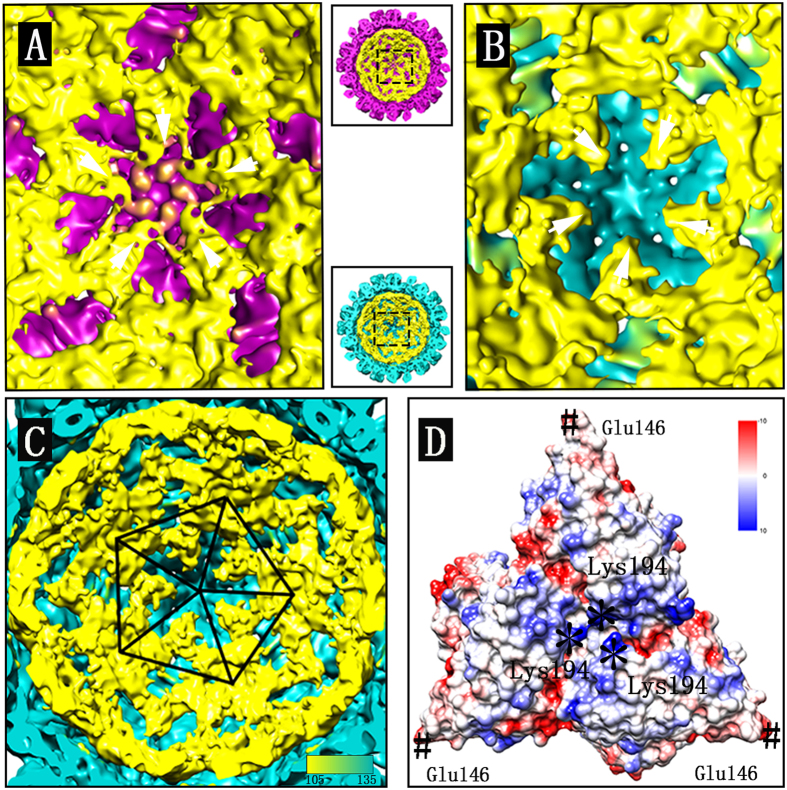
Inside views of the reconstructions. (A) Zoom-in view of the pentamer inside the reconstruction under Ca-pH7 in the middle panel between panel (A) and panel (B) (up). (B) Zoom-in view of the pentamer inside the reconstruction under EDTA-pH7 in the middle panel between panel (A) and panel (B) (low). (C) One pentamer composed of five asymmetric units is outlined inside the reconstruction under EDTA-pH7. (D) The asymmetric unit of the X-ray structure colored by Coulombic electrostatic potential shows that three Lys194 residues are located near the center of the asymmetric unit on the inner surface as marked with *, and three Glu146 residues are located at the vertices of the triangular asymmetric unit as marked with #. The genomic RNA densities close to the capsid are colored in yellow. All the density maps shown are low-pass filtered at 8 Å resolution and normalized before visualization with the contour level set at 1.8 in Chimera.
